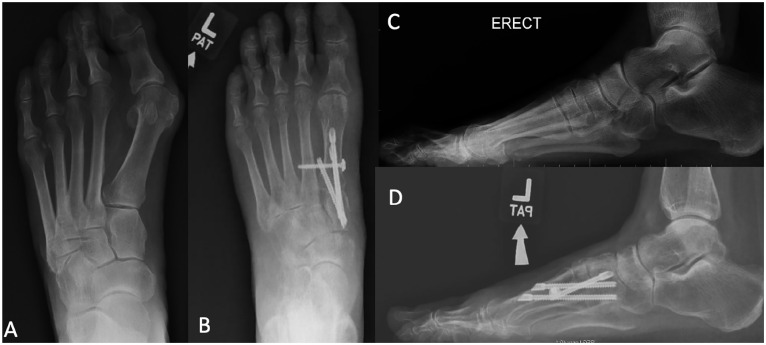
Left foot weightbearing radiographs of a patient undergoing minimally
